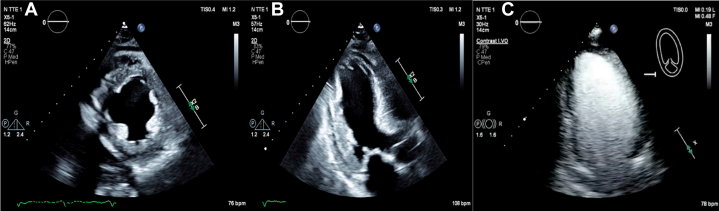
Transthoracic Echocardiogram Showing Reduced Left Ventricular Systolic Function With Regional Wall Motion Abnormality in the Left Anterior Descending Artery Territory
